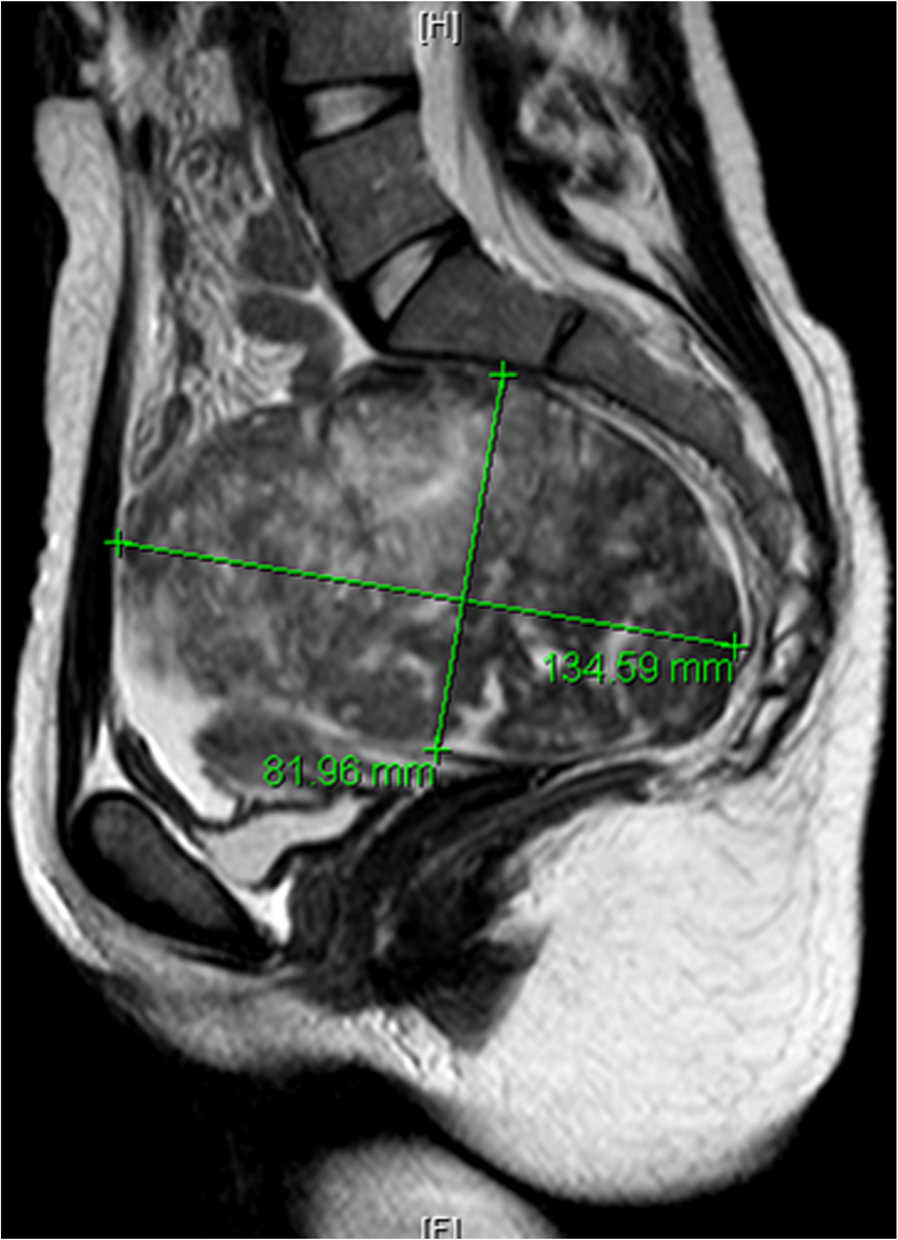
Magnetic resonance imaging scan of the pelvis demonstrating a large heterogeneous mass arising from the left ovary, extending from the sacrum to the abdominal rectus muscle.
